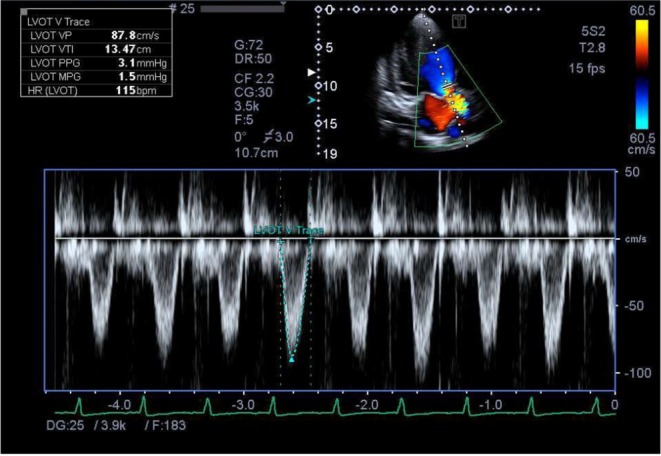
Doppler echocardiogram showing left ventricular systolic dysfunction.
